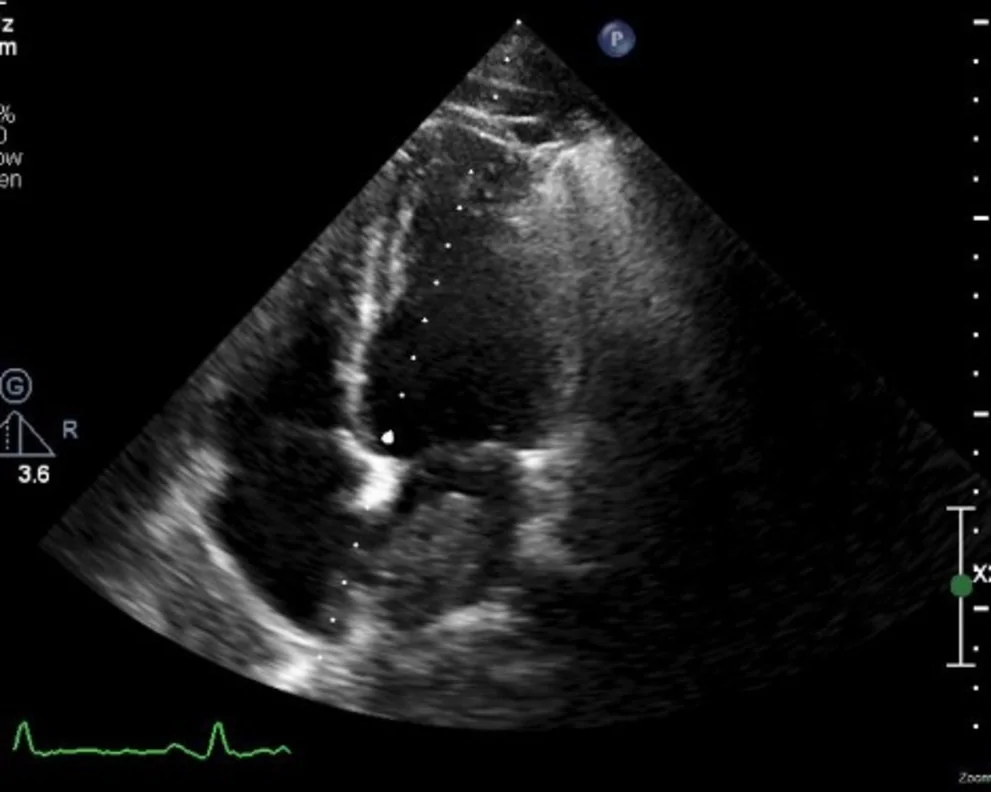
A transthoracic echocardiogram (TTE) in the 4‐chamber view, showing the left atrial mass and its significant effect on the right pulmonary veins.
